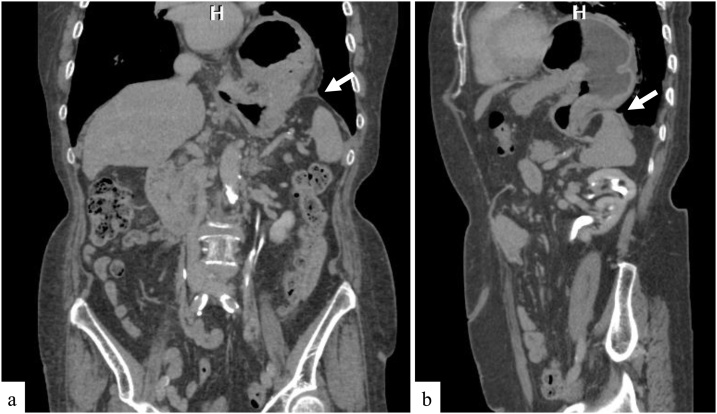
Preoperative images of the Bochdalek hernia. CT reveals herniation of the gastric corpus through the left posterior diaphragm (white arrow) in a coronal view (a) and a sagittal view (b).
